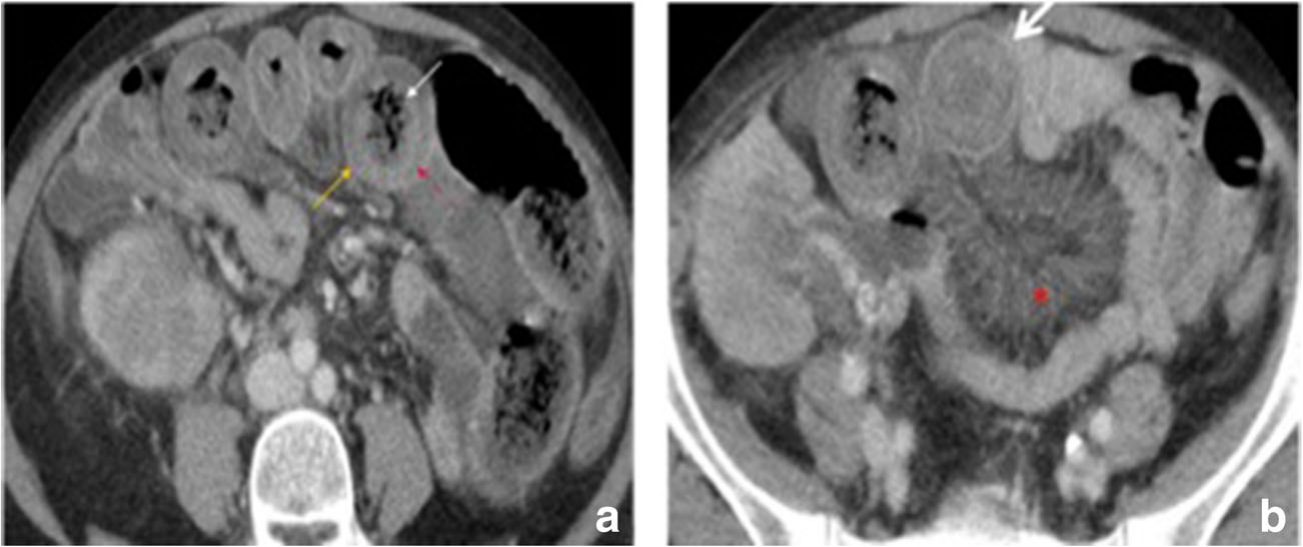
Bowel wall thickness and enhancement. a Contrast-enhanced axial CT images show a target sign (larger white arrow) in the small bowel wall due to mesenteric venous occlusion. b The vascular engorgement (*) and oedema of the bowel wall in turn lead to leakage of extravascular fluid into the bowel wall and mesentery. The resultant oedematous bowel may have a “halo” or “target” appearance due to mild mucosal enhancement (straight white arrow), submucosal and muscularis propria nonenhancement (red arrow), and mild serosal/subserosal enhancement (orange arrow). Bowel wall thickness is greatly increased measuring up to 1.5 cm
